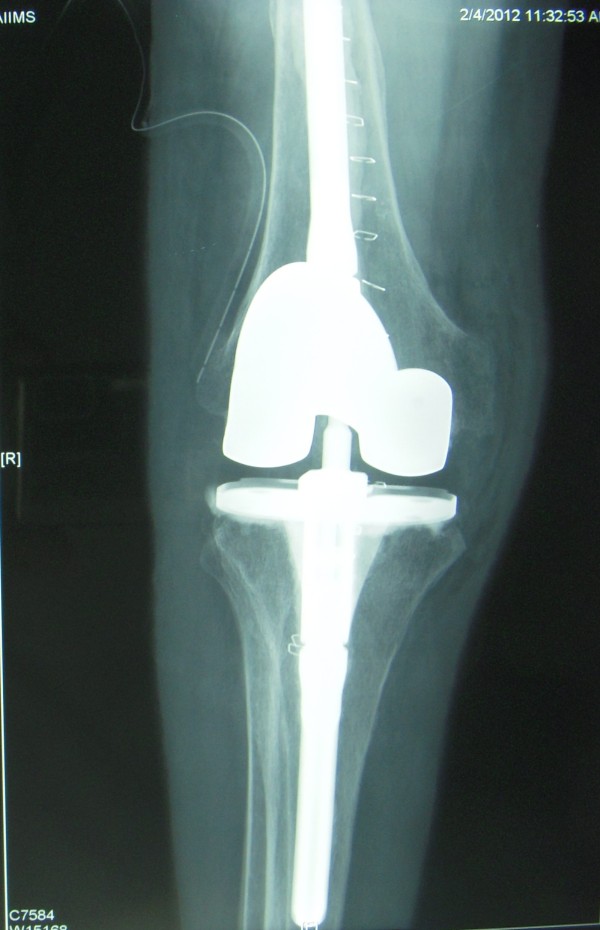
Postoperative AP radiograph of the right knee joint.
